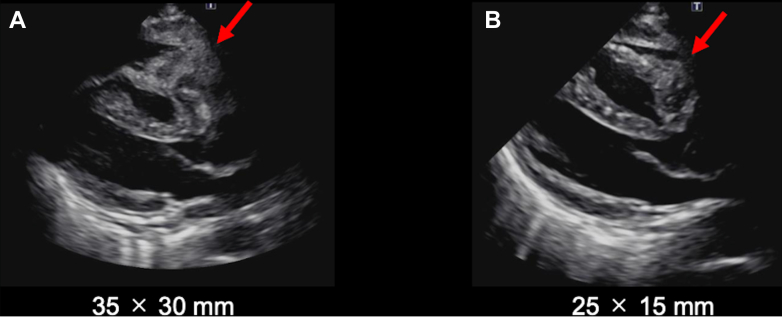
Changes in Tumor Size on Transthoracic Echocardiography The pericardial tumor (red arrows), which measured 35 × 30 mm at admission (A), decreased in size to 25 × 15 mm at discharge (B) after 2 cycles of chemotherapy.
